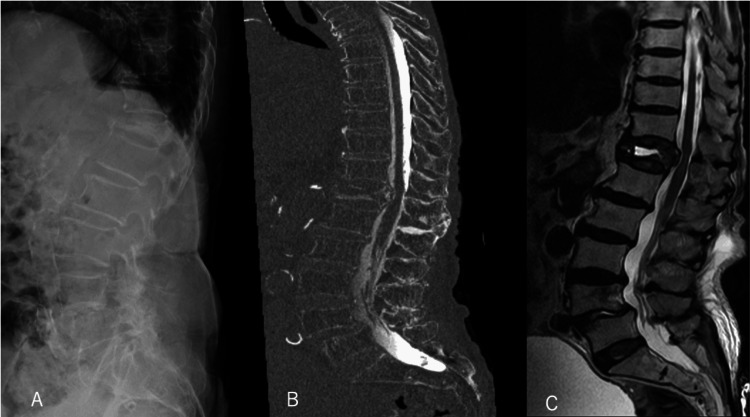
Preoperative Imaging A: Lateral radiograph showing a T12 compression fracture. B: CT myelography showing severe spinal canal stenosis. C: T2-weighted MRI showing intravertebral high signal intensity.
